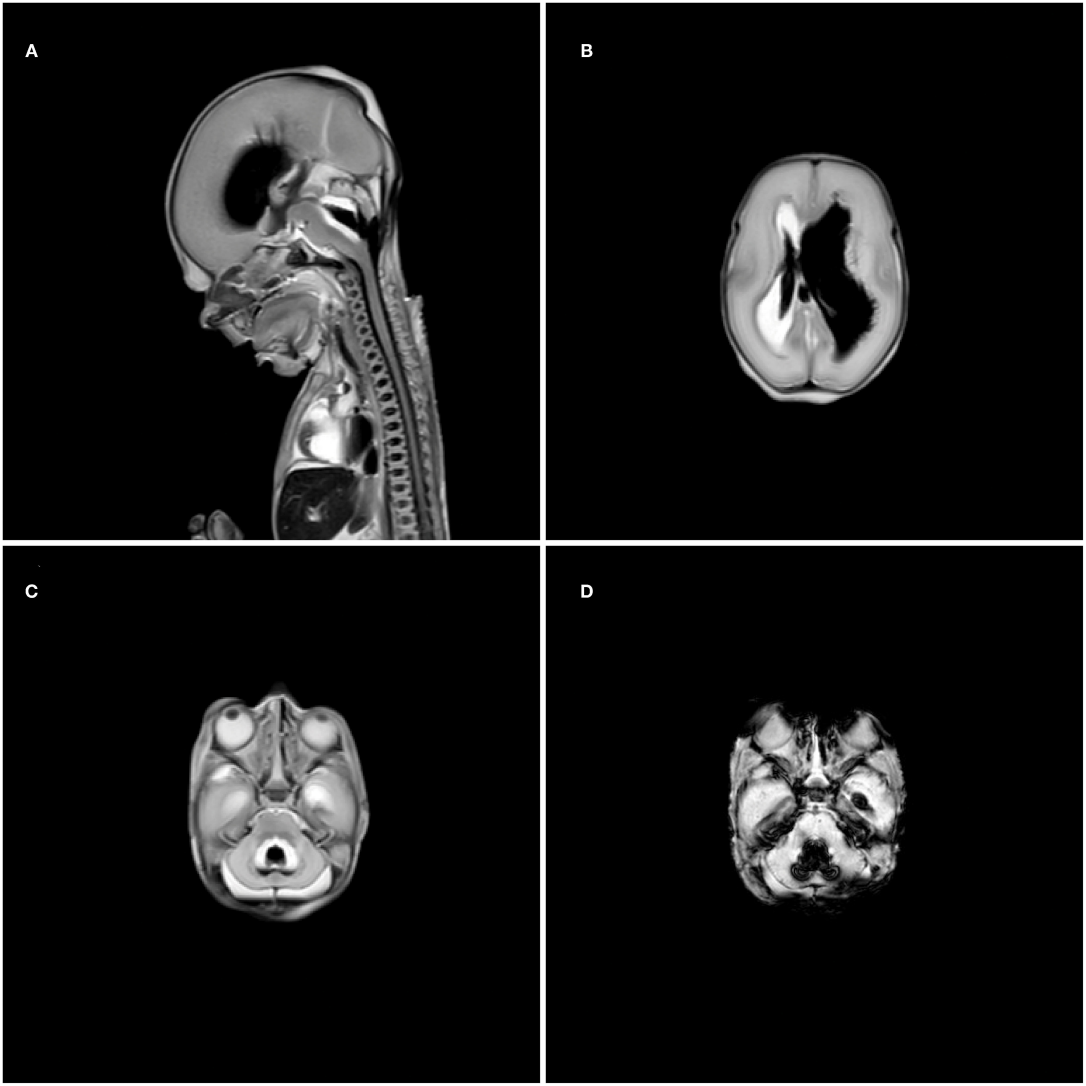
Postmortem magnetic resonance imaging showing extensive ventricular dilatation and blood in the 4^th^ ventricle in T2-weighted midsagittal plane (A), intraventricular blood in the right ventricle and ventricular dilatation of the left ventricle in T2-weighted axial plane (B), blood in the 4^th^ ventricle with cerebellar dysplasia in T2-weighted axial plane (C) and susceptibility weighted image axial plane (D) of the posterior fossa.
